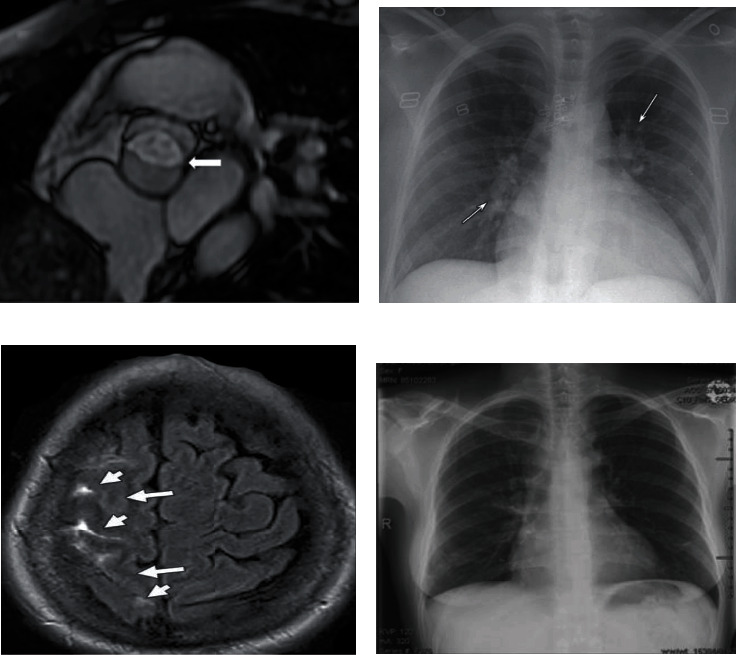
(a) In a woman, in 18 weeks of her 2nd pregnancy, the magnetic resonance appears bicuspid aortic valve (arrow) showing moderately enlarged ascending aorta up to 4.75 cm [28]. (b) Posteroanterior chest radiographs in a 22-year-old woman during and post pregnancy. At 36 weeks, the first radiograph was taken which showed an enlarged cardiac silhouette and engorged pulmonary vasculature (arrows) with apical redistribution [29]. (c) This is a case of a 24-week pregnant woman at her 30-year-old along subarachnoid hemorrhage due to eclampsia complication. Axial FLAIR MR image shows the presence of subarachnoid blood in the right posterior frontal and parietal sulci (short arrows) and subtle edema covering the underlying cortex (long arrows) [30]. (d) Peripartum cardiomyopathy showed in a pregnant woman by chest radiography [31].
